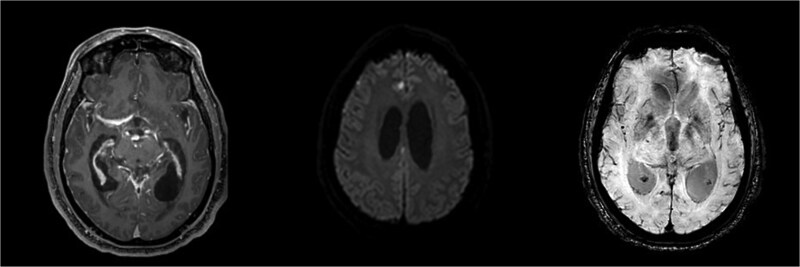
MRI of the brain revealing ventriculitis, hydrocephalus, microhemorrhages, and small punctate infarcts in the inferior frontal lobes and right paramedian frontal lobe.
